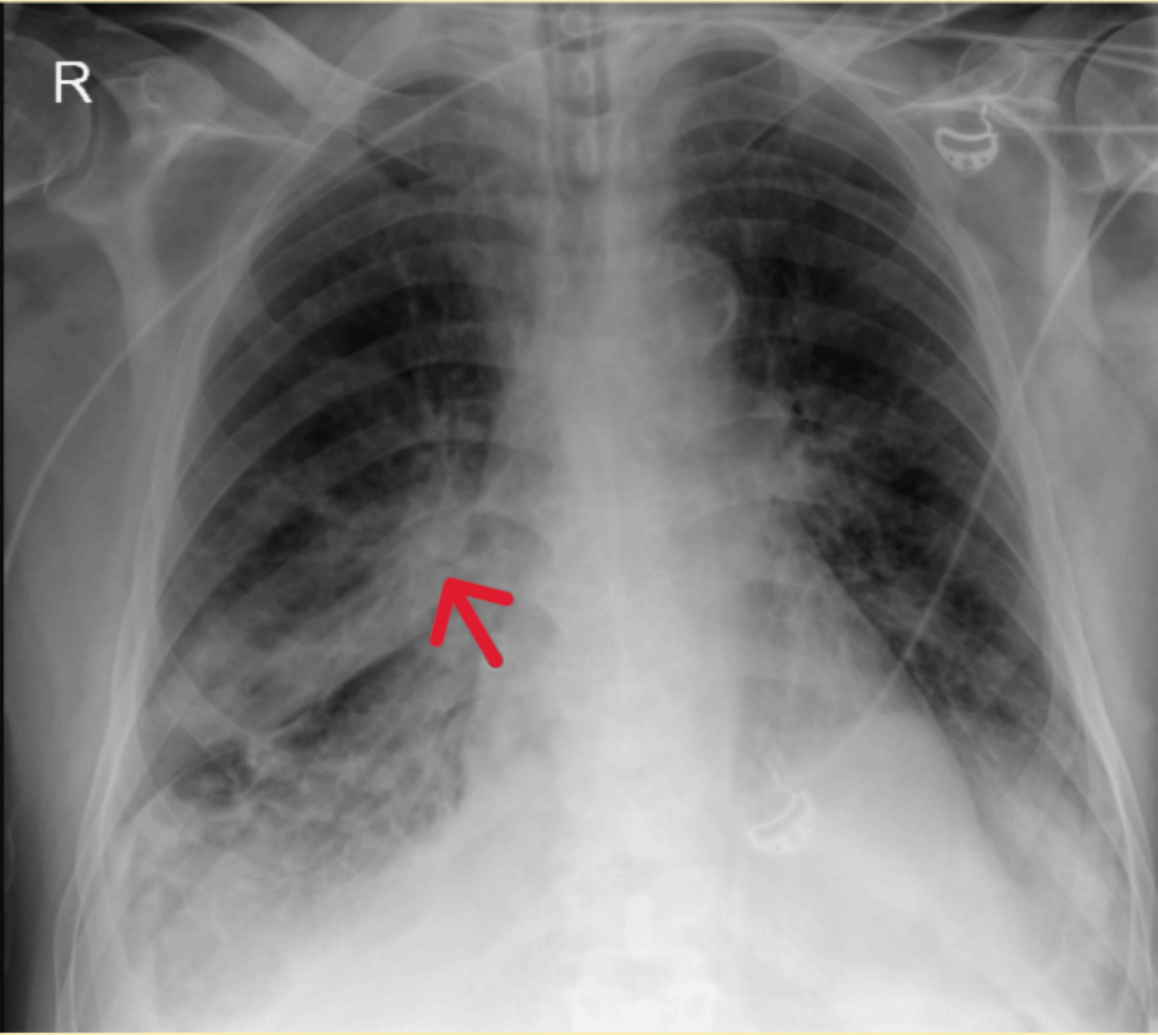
Chest X-ray with opacity of the right middle and lower lobe (red arrow)
